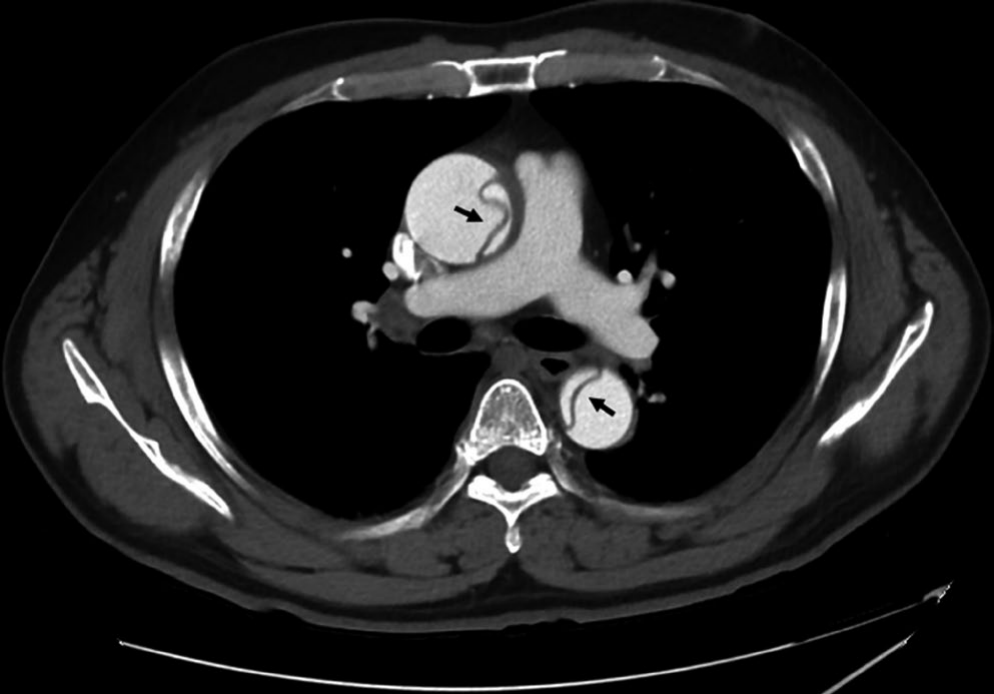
Computed tomography imaging showing the intimal flap in the aorta diagnosed as acute Stanford type A aortic dissection
